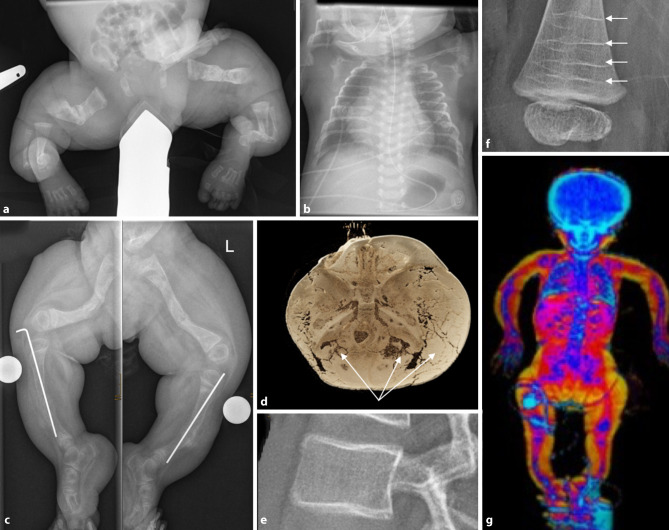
Osteogenesis imperfecta. a Multiple congenital fractures, b slender, gracile ribs, c orthopedic pin stabilization of the tibia. d Three-dimensional reconstruction of skull base (by computed tomography). Visibility of multiple wormian bones (*arrows*). e Teenager with mild form of osteogenesis imperfecta (OI). Cortical framing after bisphosphonate therapy, f (bisphosphonate-induced) metaphyseal Zebra lines (*arrows*), g dual-energy X‑ray absorptiometry (DXA) whole-body image (short stature, extremity bowing, macrocephaly). Please note this is the same child as in a, b (as a newborn), now 3 years old
